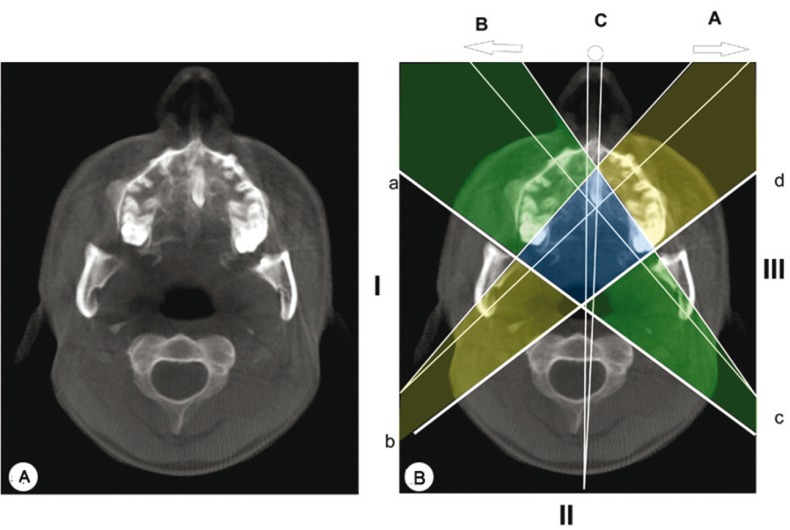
A) A primary reconstruction from the CBCT showing single midline supernumerary in the paramedian region parallel to the midpalatal suture; B) Path of x-rays in a convention RPR, origin to ARC (yellow), the diamond shaped region (blue), ARC to terminal position (green).
